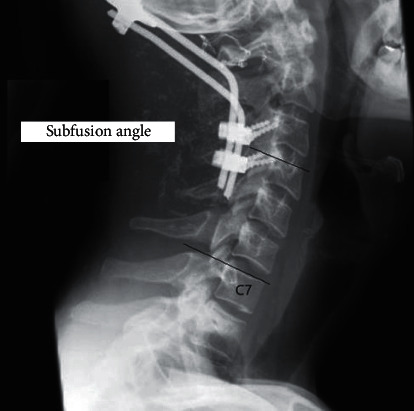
Lateral roentgenogram following POCF showing the subfusion angle measurement.
